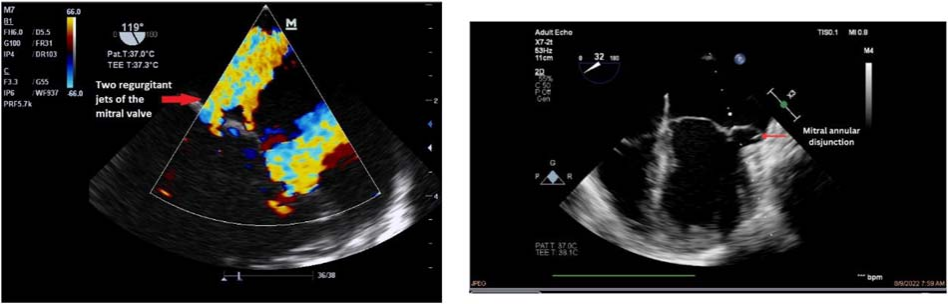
Preoperative echocardiography of patient 1.
